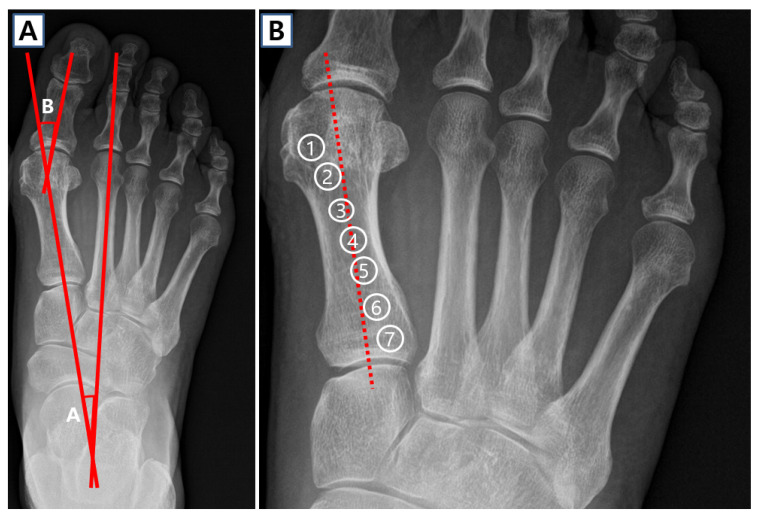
Radiographic parameters of hallux valgus. (A) The IMA (angle A) was measured as the angle between a line bisecting the second metatarsal shaft and another line connecting the center of the first metatarsal head to the center of its proximal articular surface. The HVA (angle B) was defined by the angle created at the intersection of the longitudinal axis of the first metatarsal—drawn from the center of its head to the center of its proximal articular surface—and the diaphyseal axis of the proximal phalanx of the great toe. (B) The tibial sesamoid position, relative to the longitudinal axis of the first metatarsal, was classified from grade I to VII according to the system described by Hardy and Clapham. IMA: intermetatarsal angle; HVA: hallux valgus angle.
